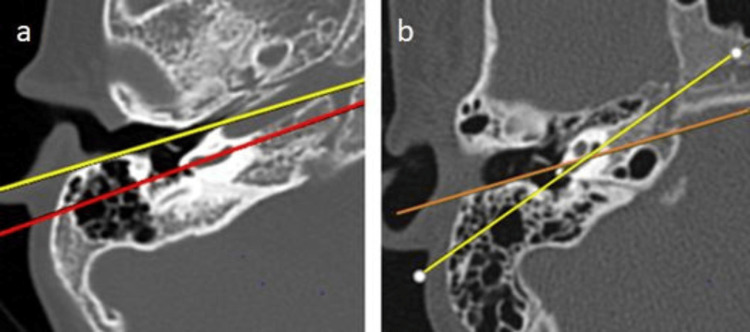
(a,b) Relation between the posterior external auditory canal and the long axis of the basal turn line HRCT of the temporal bone showing that (a) the line along the posterior wall of the external auditory canal (yellow line) and the line along the long axis of the basal turn of the cochlea (red line) are parallel and (b) the line along the posterior wall of the external auditory canal (red line) and the line along the long axis of the basal turn of the cochlea (red line) are intersecting medially. HRCT: high-resolution CT
